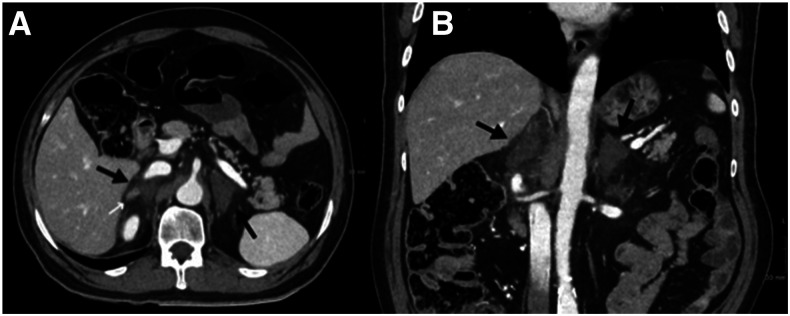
Axial (A) and coronal (B) images of abdominal CT scan with IV contrast. A marked increase in the dimensions of both adrenal glands with frayed edges, and with a general hypodense appearance (black arrows) is identified. A more attenuating pseudo-nodular image is identified within the right adrenal gland, which probably corresponds to undamaged tissue (white arrow). In addition, they are also associated with moderate inflammatory changes due to contiguity, with trabeculation of the peri-adrenal mesenteric fat. These findings are compatible with bilateral adrenal hematomas.
